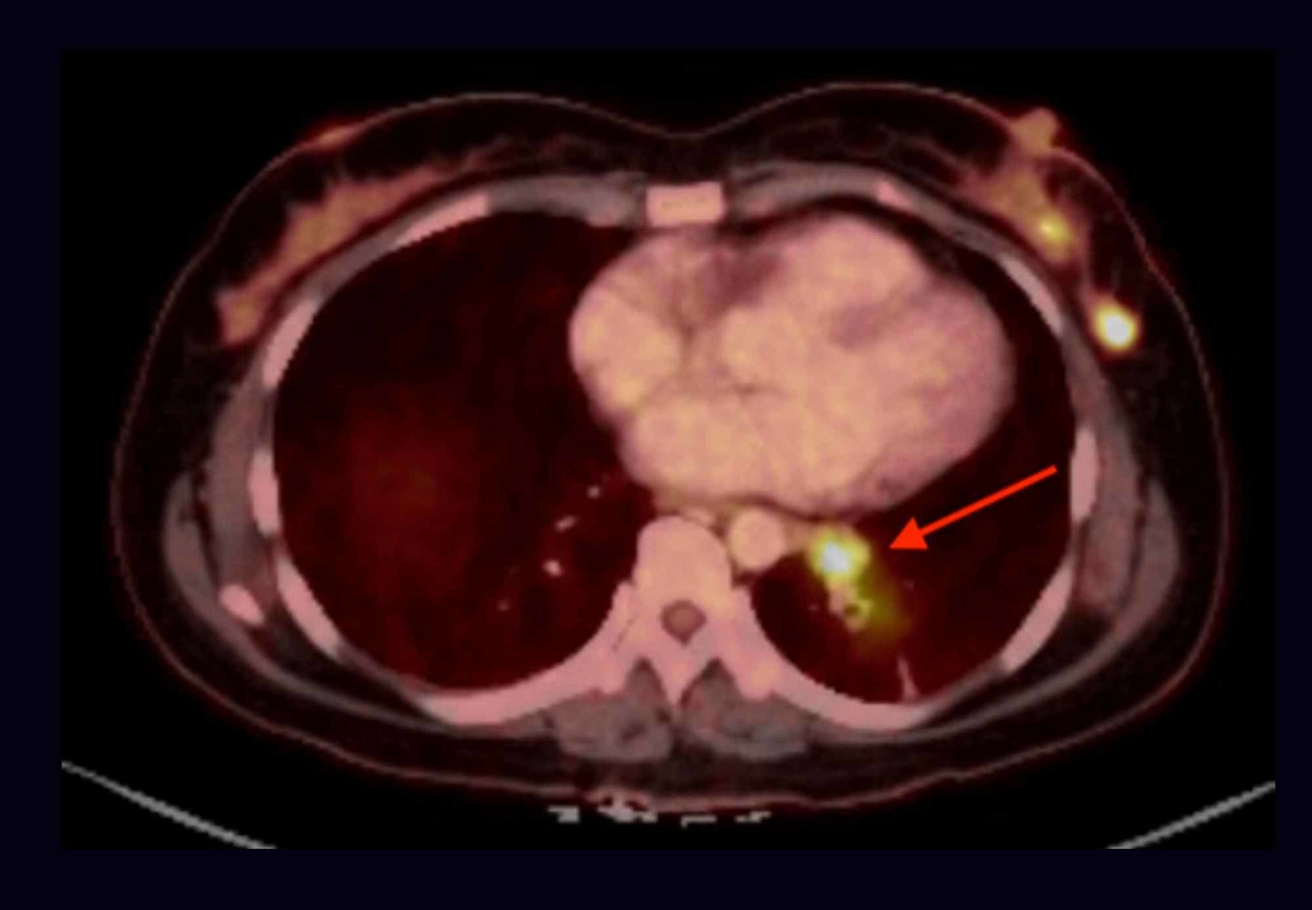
PET/CT showing FDG avid 2.9 x 1.8 x 1.8 cm hilar LN at station 11L-12L (red arrow) metastatic atypical carcinoid (max SUV 12.6) PET/CT: positive emission tomography/computed tomography; FDG: fluorodeoxyglucose; cm: centimeter; LN: lymph node; SUV: standardized uptake value
